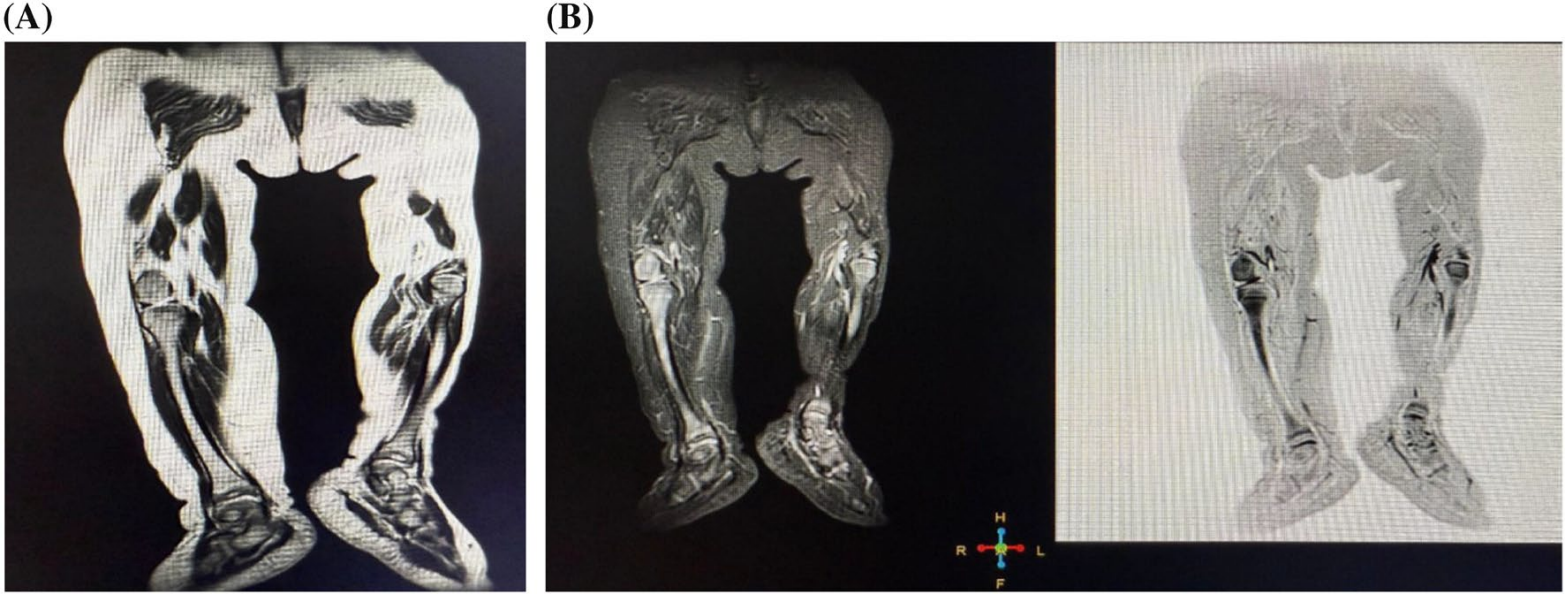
(A) Coronal T2‐weighted MRI of both lower legs demonstrating diffuse fat infiltration in the right lower leg. (B) Coronal STIR images showing appropriate fat suppression. No dilated vessels or varicosities are observed in the subcutaneous plane, and bone marrow signal intensity remains normal.
